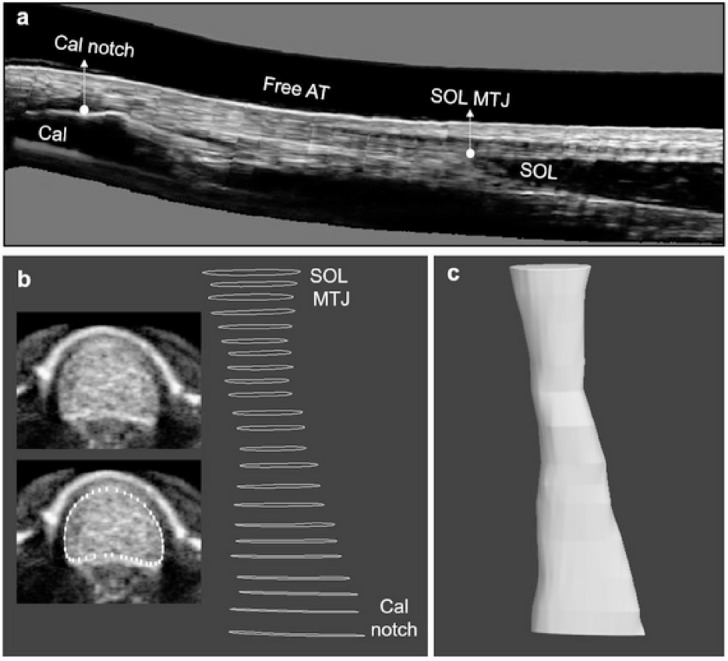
Measurement of free Achilles tendon (AT) length, cross-sectional area (CSA) segmentation, and volume reconstruction from a representative participant. (a) Sagittal plane re-slices of a reconstructed three-dimensional (3D) ultrasound image of the free AT. Two anatomical landmarks [calcaneal (Cal) notch and soleus muscle–tendon junction (SOL MTJ)] were identified and segmented on 3D AT images to determine tendon length. (b) Representative transverse ultrasound image of the AT showing the manual digitization of the CSA. The CSA of the free AT was manually digitized from transverse images at 2 mm intervals extending from the Cal notch to the SOL MTJ. (c) The 3D volume of the free AT was reconstructed from the segmented CSAs using a surface interpolation algorithm implemented in Stradwin software (v6.03, https://mi.eng.cam.ac.uk/Main/StradWin).
